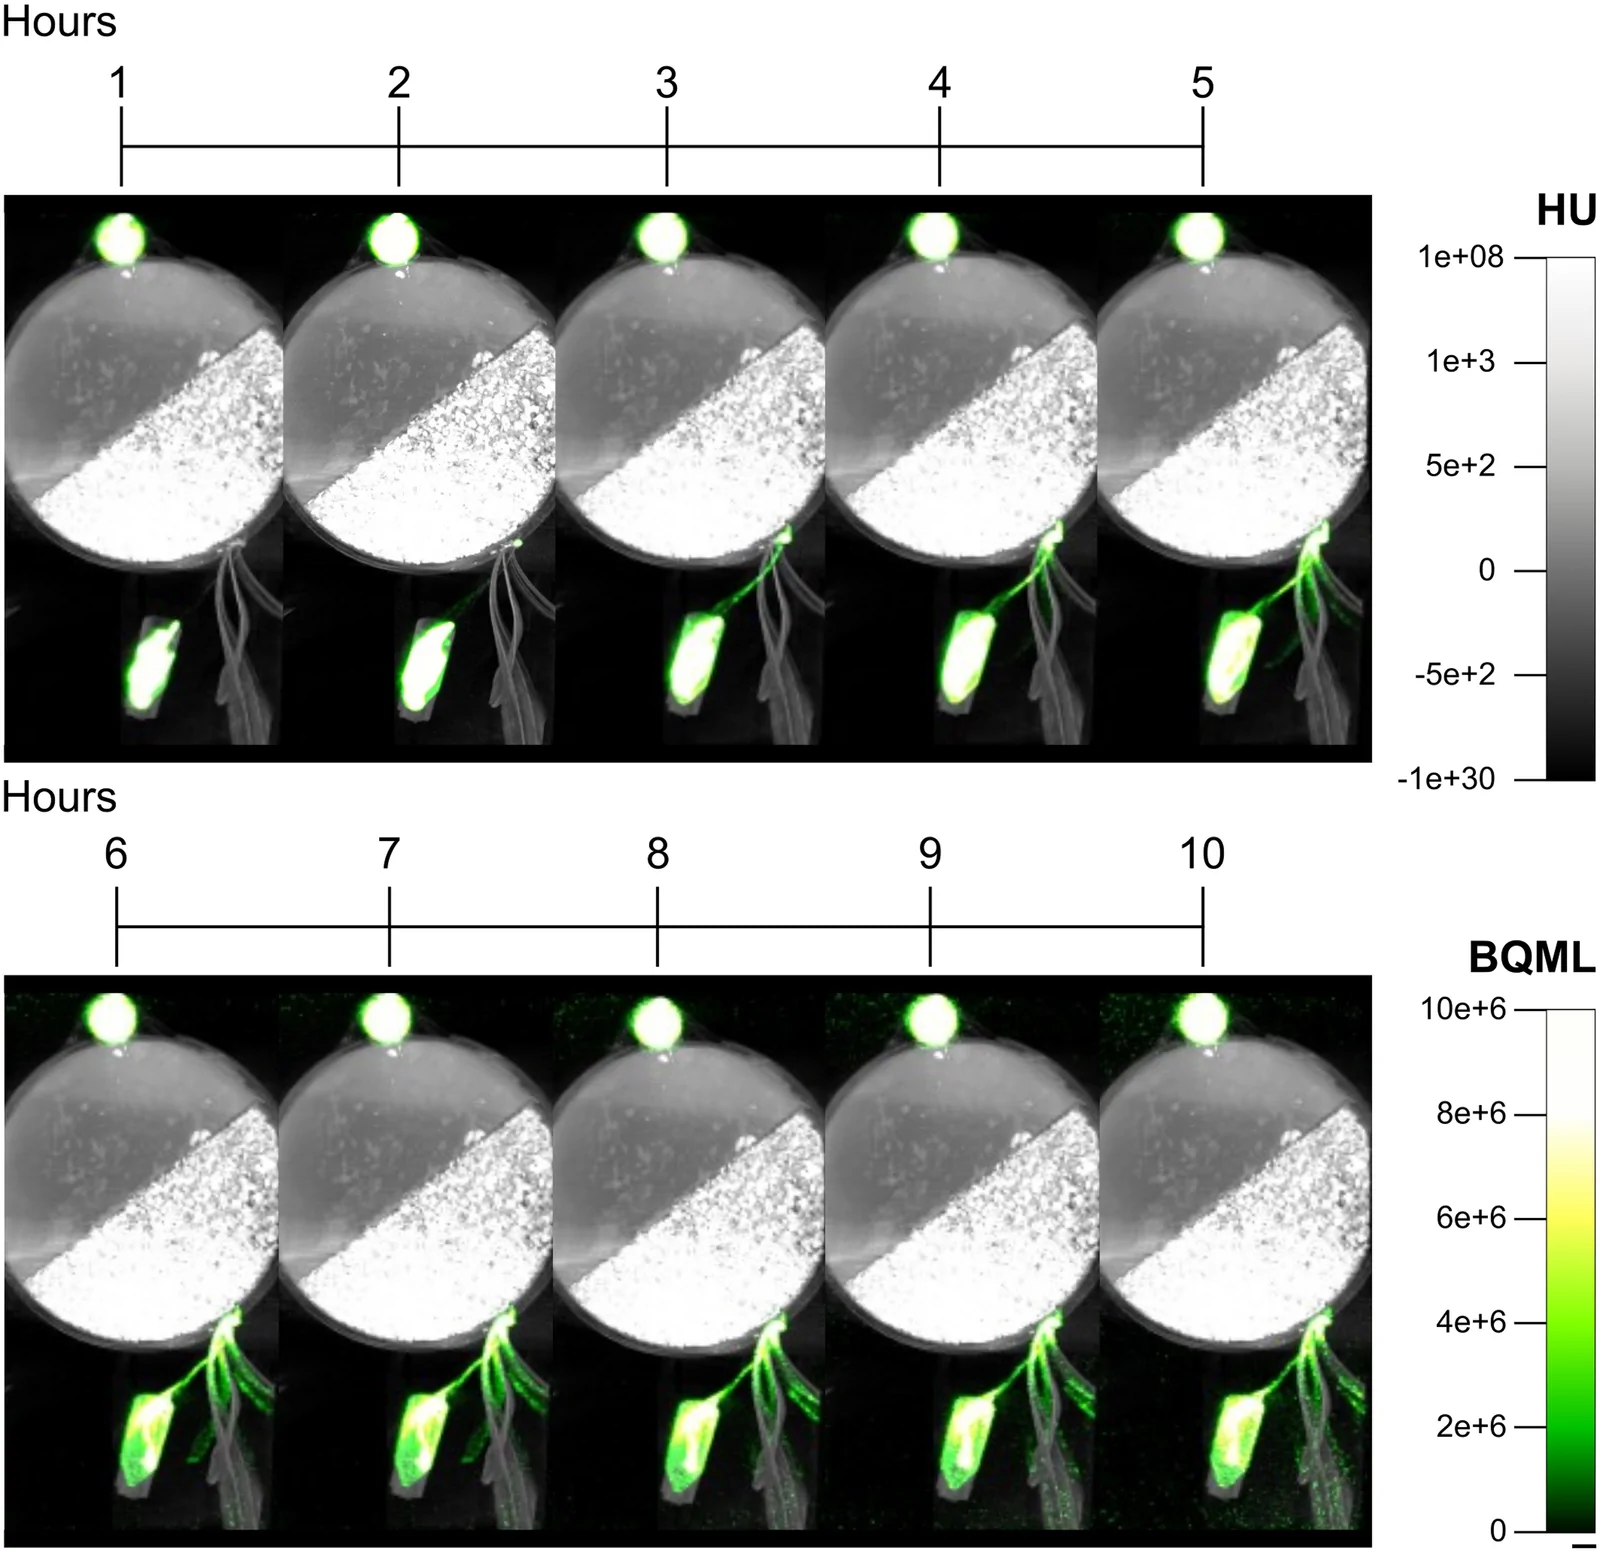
Maximum intensity projection (MIP) views of a PET/CT hourly time course of the uptake of 11-[^18^F]fluoroundecanoic acid by a leaf of *Plantago lanceolata* immersed in a solution of the tracer. The use of PET-labelled fatty acid substrates has been demonstrated elsewhere (Cai *et al.*, 2016). No uptake was observed via the root, which was also dipped in a separate vial containing 20 MBq of the same tracer (top of image).
